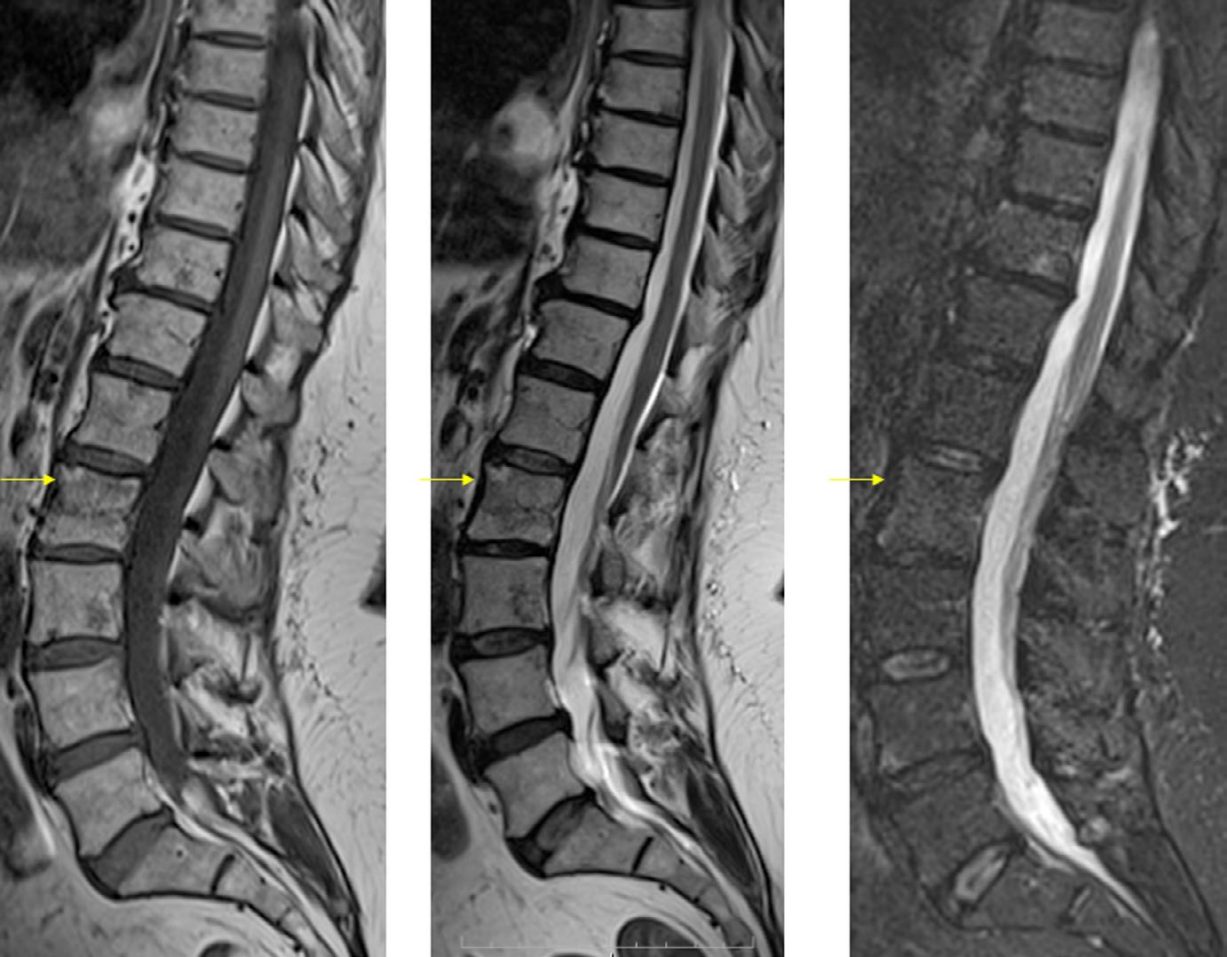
Follow‐up MRI shows almost complete disappearance of bone marrow abnormalities
